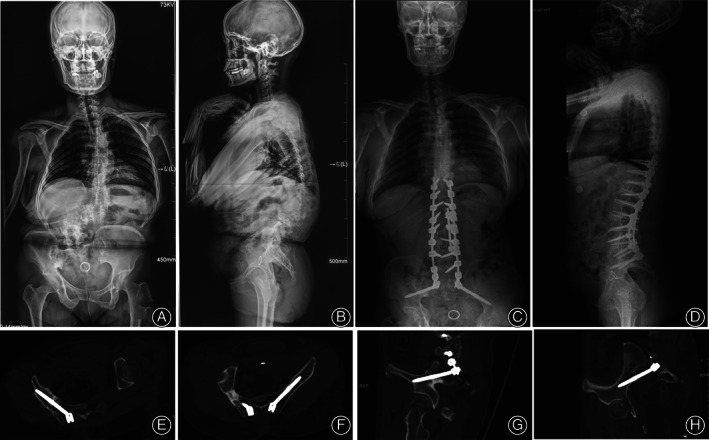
Typical case 1 managed by ball tip technique. A 44‐year‐old female presented severe congenital thoracolumbar deformity with a morphological abnormality of right sacroiliac joint, in which the hypertrophy is obvious under the overload stress. Also, the pelvis is asymmetrical with the right side being anteriorly rotational (A, B). The anomaly made the S2AI screw insertion by conventional free hand technique to be difficult. Surgeon conducted the ball tip technique to solve this problem perfectly. Postoperative X‐rays showed the satisfactory correction and good sagittal and coronal realignments with a sacropelvic fixation (C, D). Postoperative CT scan indicated the perfect positions of S2AI screws bilaterally in the axial and sagittal plates of screws without any cortex breach (E–H).
